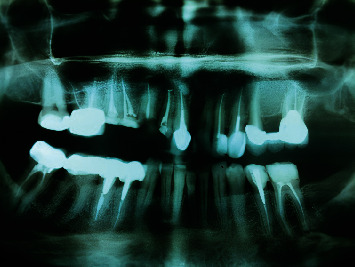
Panoramic radiographic view of patient's teeth.
